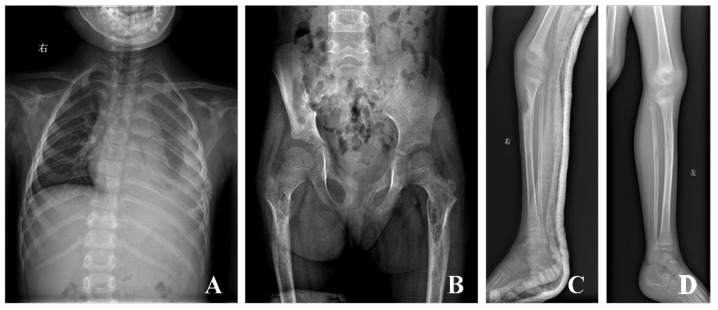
(A) X-ray reveals multiple osteolysis, including bilateral clavicle, bilateral scapula, the 4th, 5th, 9th, 10th right ribs, the 2th and 6th-10th left ribs, the third, fourth and fifth lumbar vertebra, (B) right ilium, sacrum, bilateral femur, (C) right tibia, and (D) left tibia was normal as control.
